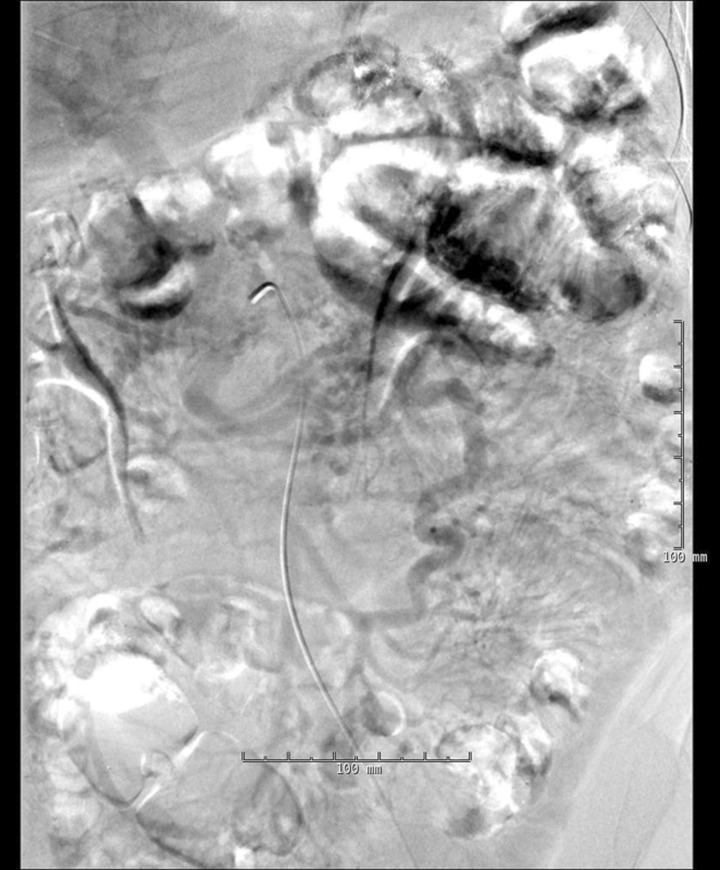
Computed tomography angiography of the superior mesenteric artery was filmed into the portal venous phase. There was filling of the ileocolic vein, as well as of the jejunal branches. At the confluence of the jejunal branches with the ileocolic vein, there was an abrupt occlusion at the ligament of Treitz, suggestive of the operative finding of volvulus. The normal expected proximal most portion of the superior mesenteric vein, along with its drainage into the splenic vein, is absent.
